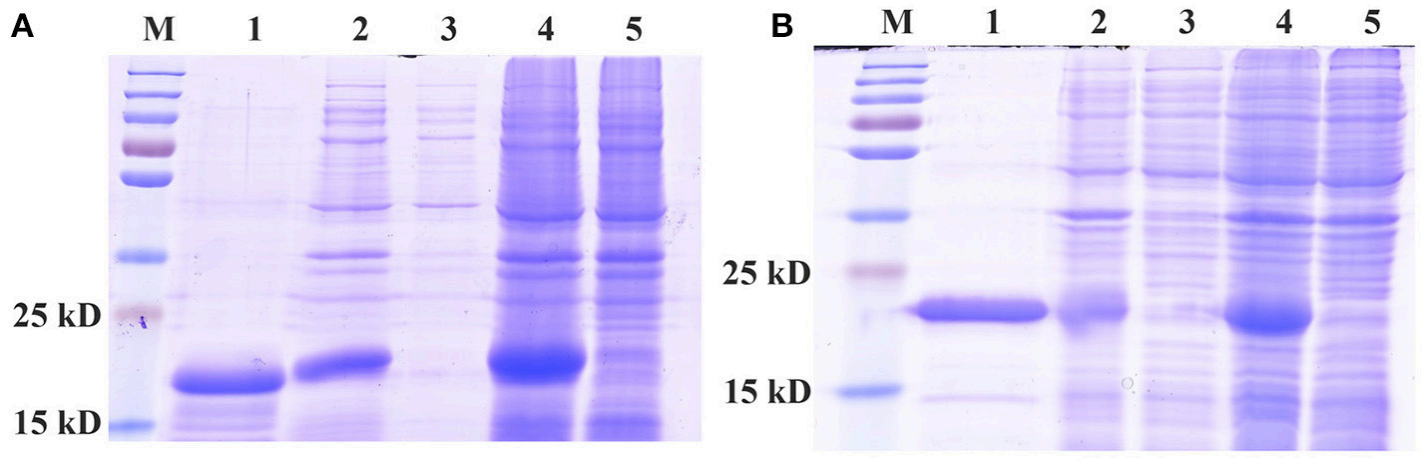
SDS-PAGE analyses of expressed recombinant of CpunPBP2 (A) and CpunPBP5 (B). M, marker protein; 1, purified fusion protein; 2, inclusion body of induced cells; 3, supernatant from ultrasound treated cells; 4, IPTG induced *E. coli* pET30a (+)/CpunPBPs transformed BL 21(DE3) cells; 5, Non-induced pET30a (+)/CpunPBPs transformed BL 21(DE3) cells.
